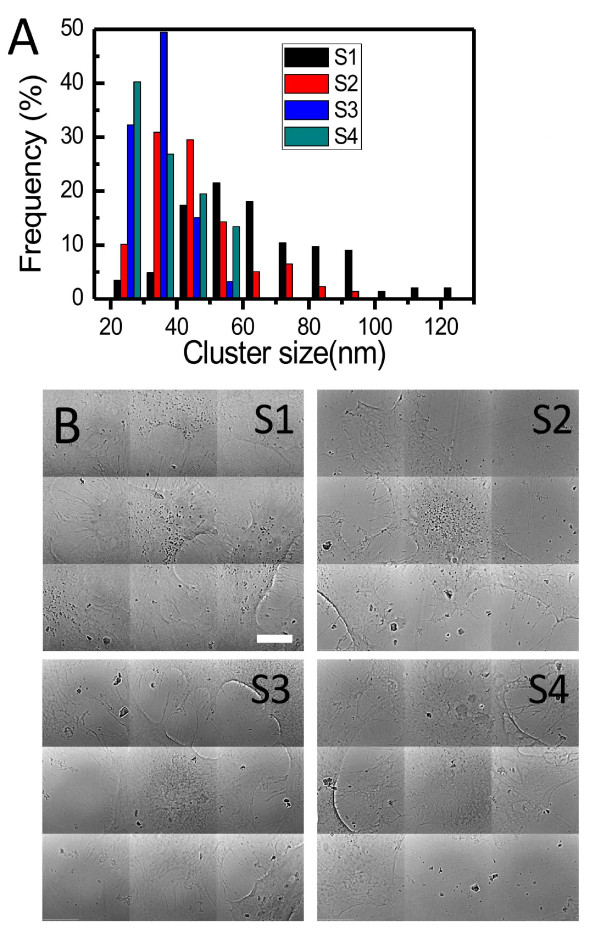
Quantitative analysis of the uptake of HeLa cells by transmission x-ray microimages. Results similar to those of Figure 10, for HeLa cells. Bar: 5 μm.
